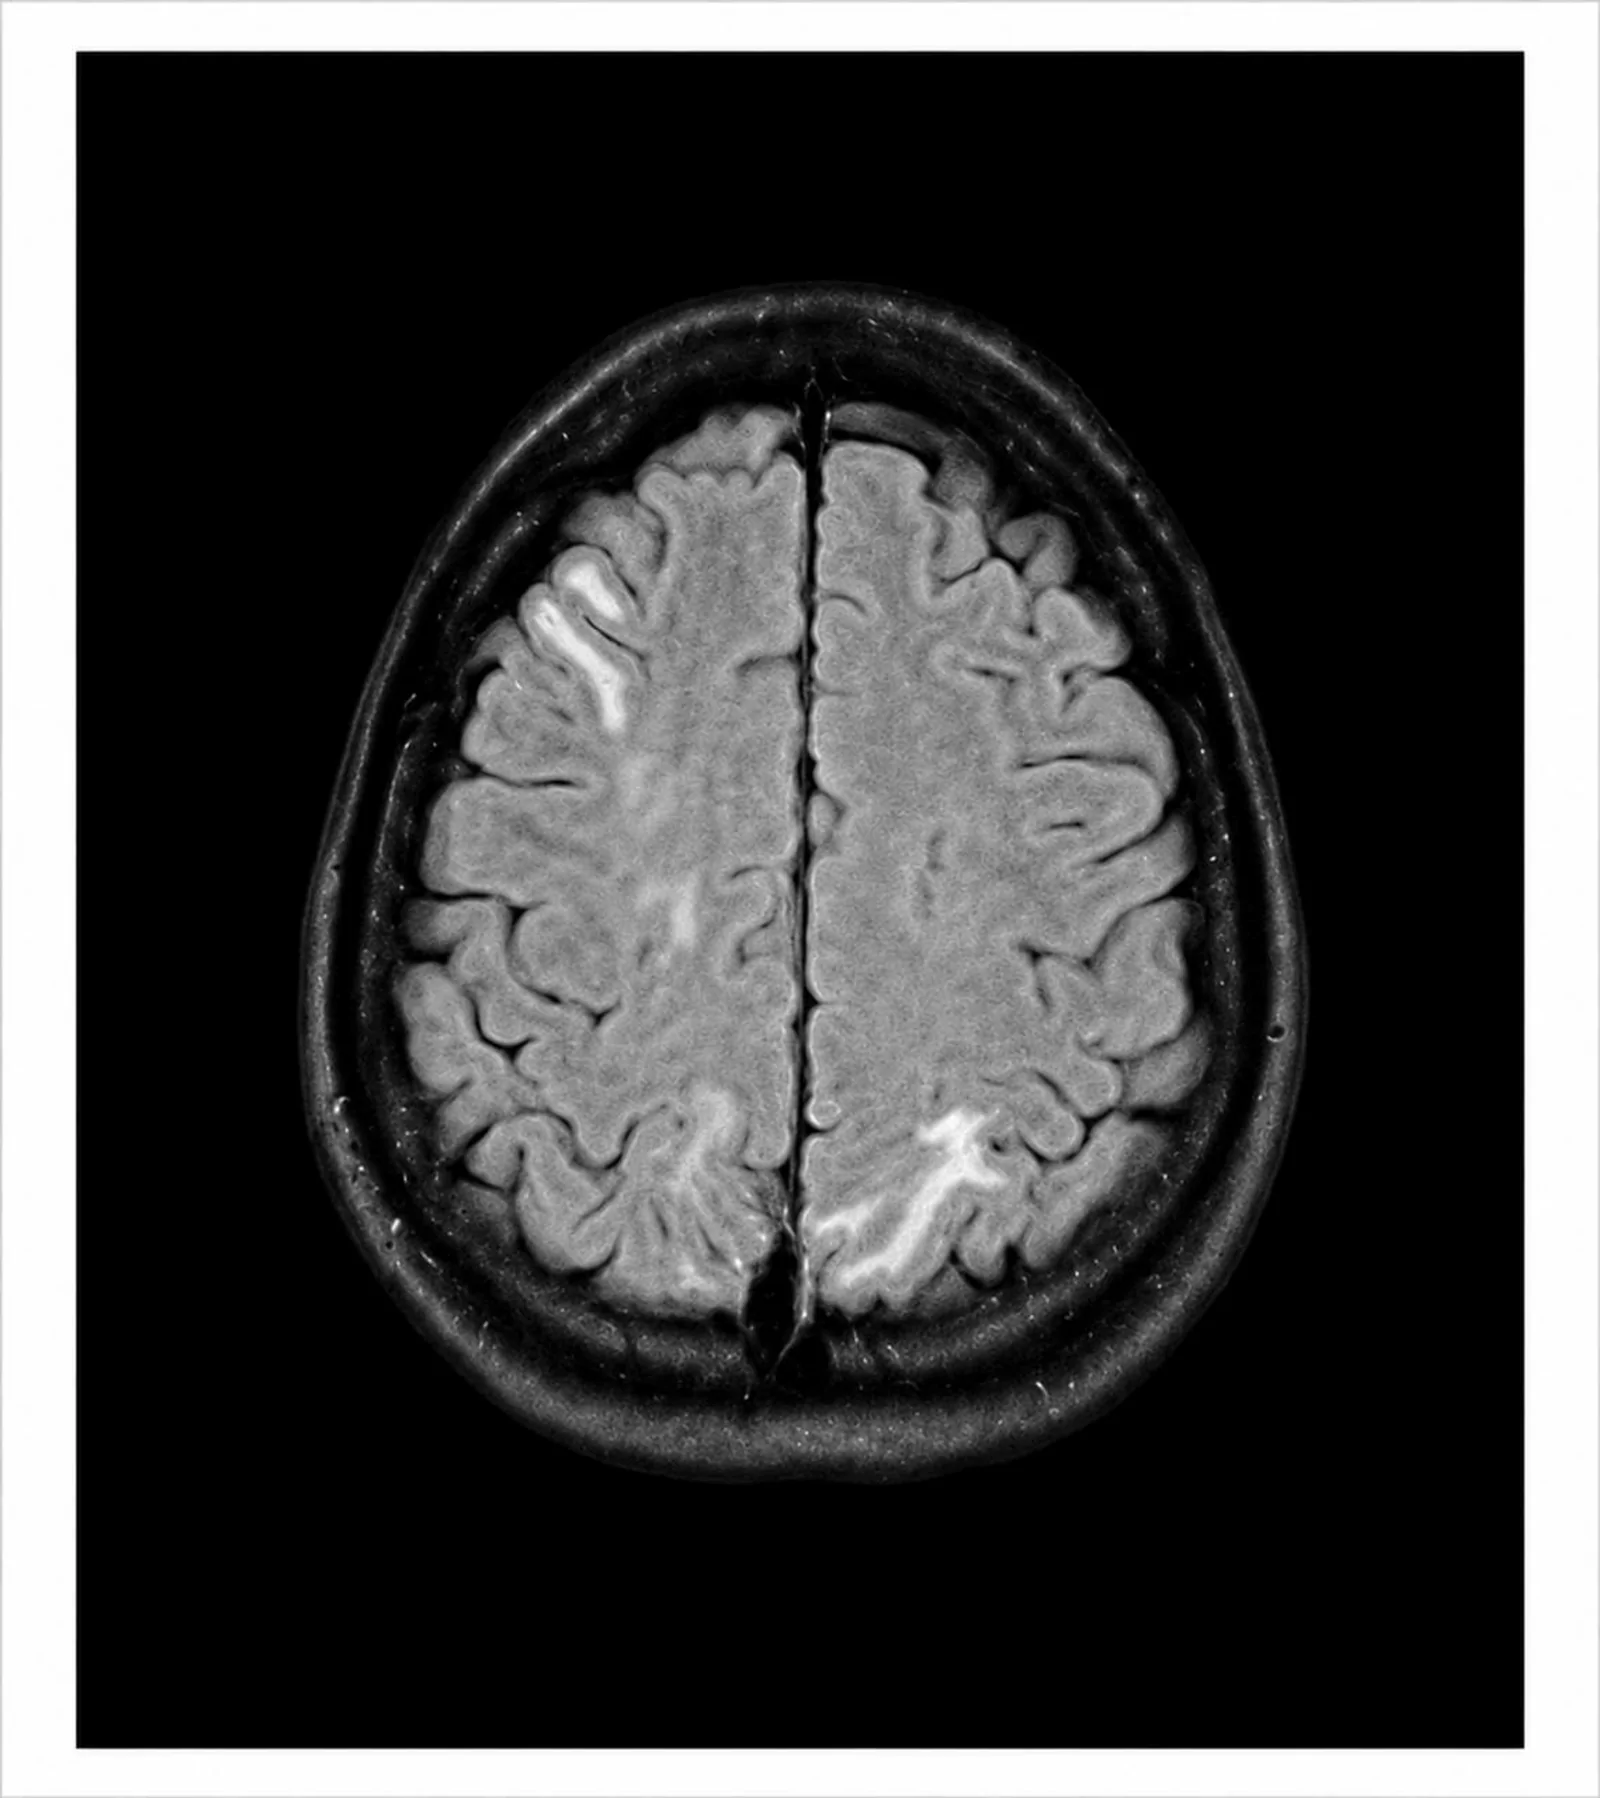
Brain magnetic resonance imaging (MRI) Axial T2-weighted MRI demonstrates bilateral asymmetric cortical and subcortical hyperintense signal abnormalities involving the parieto-occipital regions, consistent with vasogenic edema characteristic of posterior reversible encephalopathy syndrome (PRES).
